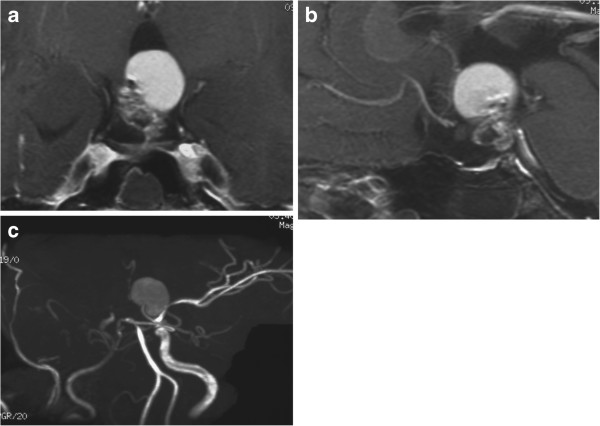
Case 2. Coronal (a) and sagittal (b) head MR images with contrast medium showing a dumbbell-shaped, partially solid and partially cystic tumor extending from the suprasellar cistern to the third ventricle. MR angiogram showing showed severe stenosis of the right ICA in the cavernous portion, and both A1 and M1 were only faintly visualized (c).
